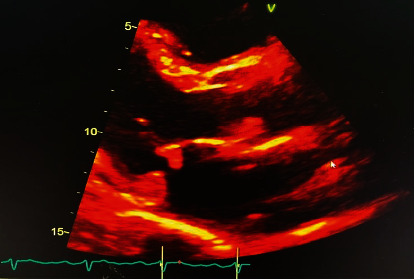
Echocardiogram demonstrating moderate-size aortic valve and moderate-size mitral valve (anterior leaflet) vegetations.
